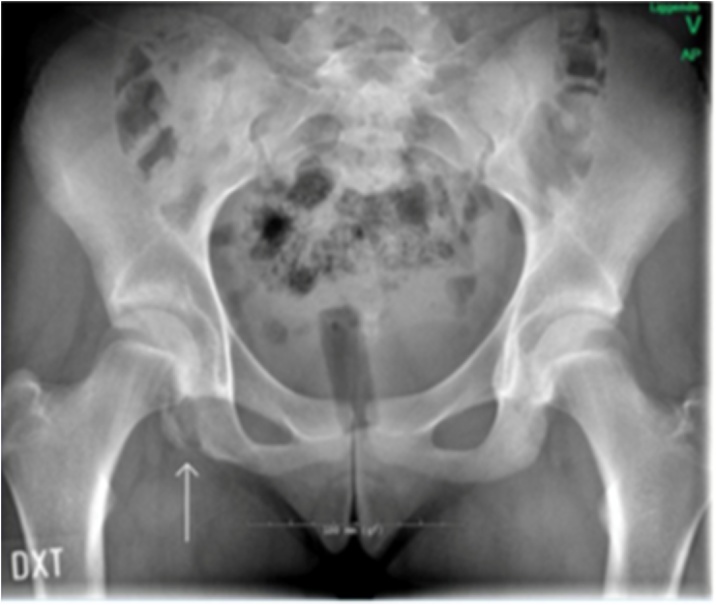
X-ray taken 6 months after symptom debut. Arrow show fracture line at the right ischial tubercle. Displacement was less than 10 mm.
